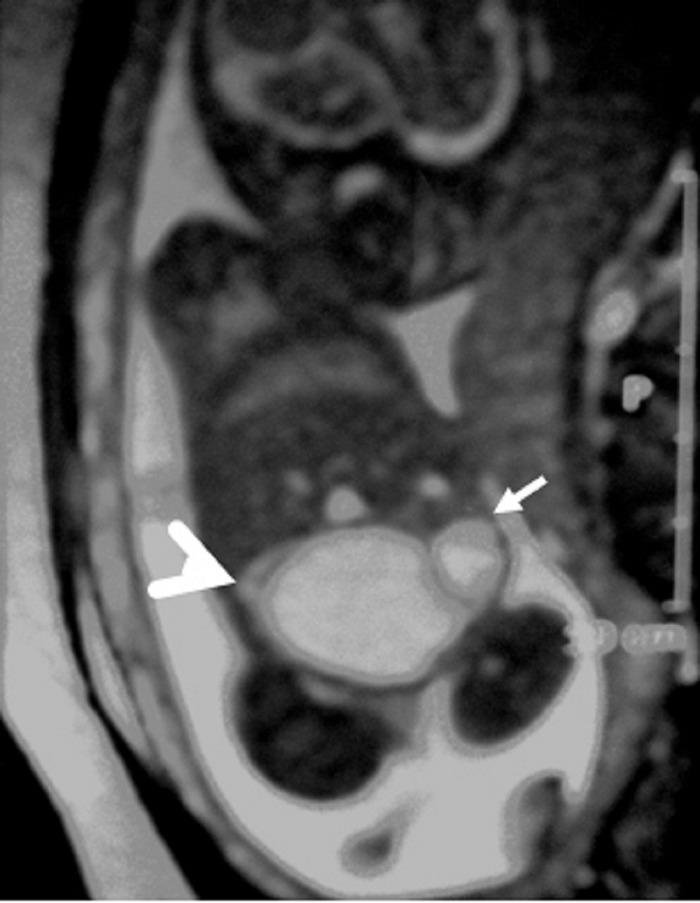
Figure 3: Fetal MRI showing type IV teratoma (big arrow head) and the white arrow demonstrates hydrometrocolpos.
